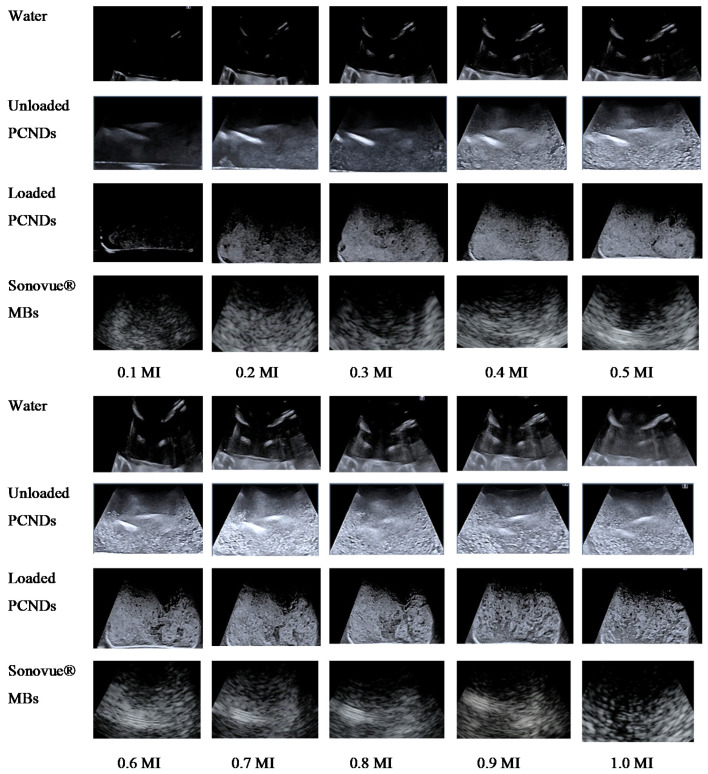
Ultrasound images of water (negative control), unloaded PCNDs, 10 mg PEG-coated SPION-loaded PCNDs in comparison to Sonovue^®^ as a positive control at MI (0.1 to 1) on 3.5 MHz frequency.
